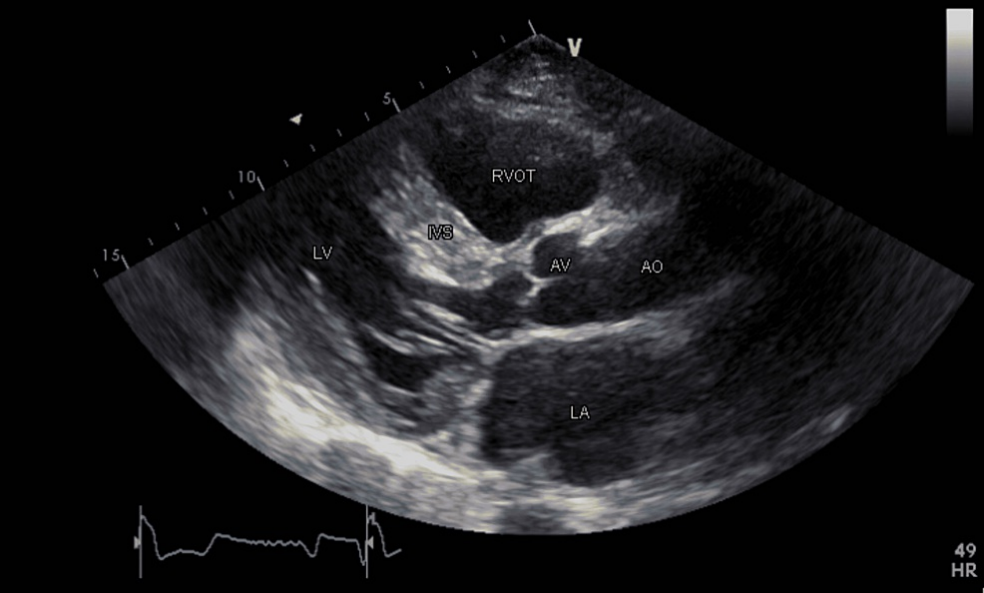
Parasternal long-axis view showing interventricular septal hypertrophy and dilated left atrium RVOT: right ventricle outflow tract; AO: aorta; AV: aortic valve; LV: left ventricle; LA: left atrium; IVS: interventricular septum
